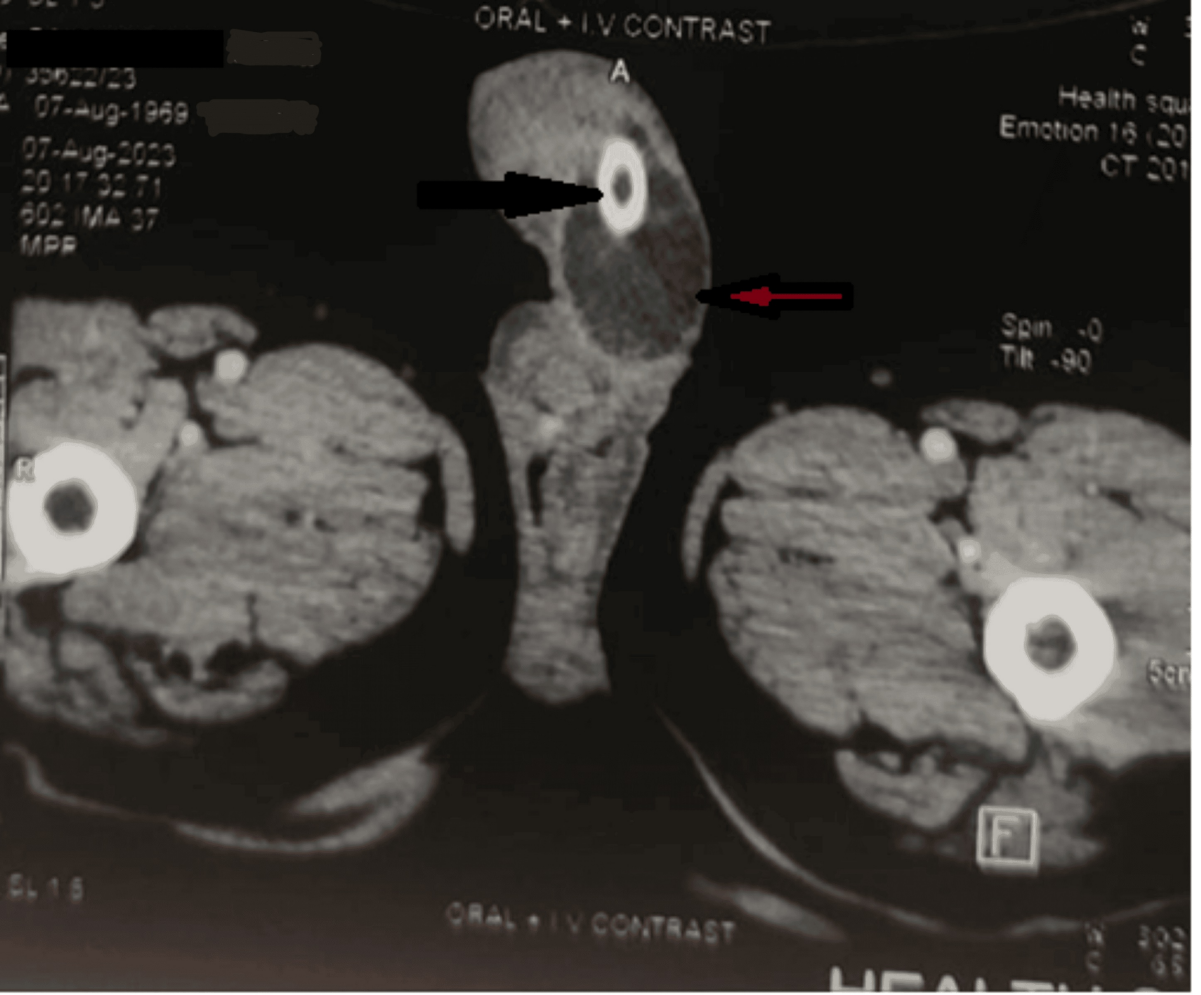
CT film showing drain in posterior urethral (black arrow) with periurethral collection (red arrow)
